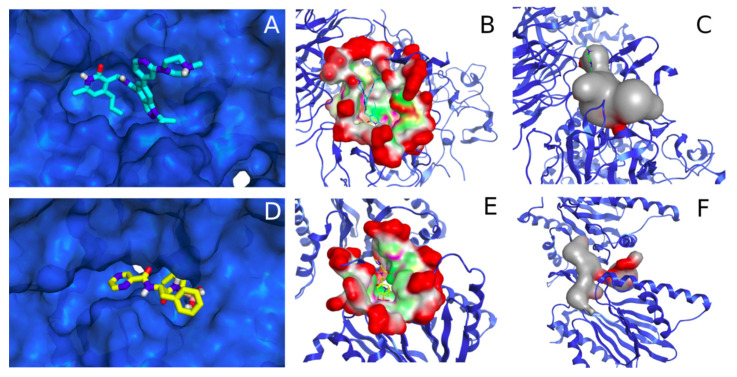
Binding pockets in EZH2 and P20S, respectively. The top row refers to EZH2’s pocket while the bottom row refers to P20S; (A,D) X-ray ligand in the binding pocket (GSK343 in cyan and bortezomib in yellow); (B,E) ligand in the binding pocket whose surface is colour-coded according to exposed (red), hydrophobic (green), and polar (pink) regions; (C,F) volume contained within the boundaries of the pocket cavity, hydrophilic (red), hydrophobic (grey). The detected pockets are defined in Supporting Table S1.
